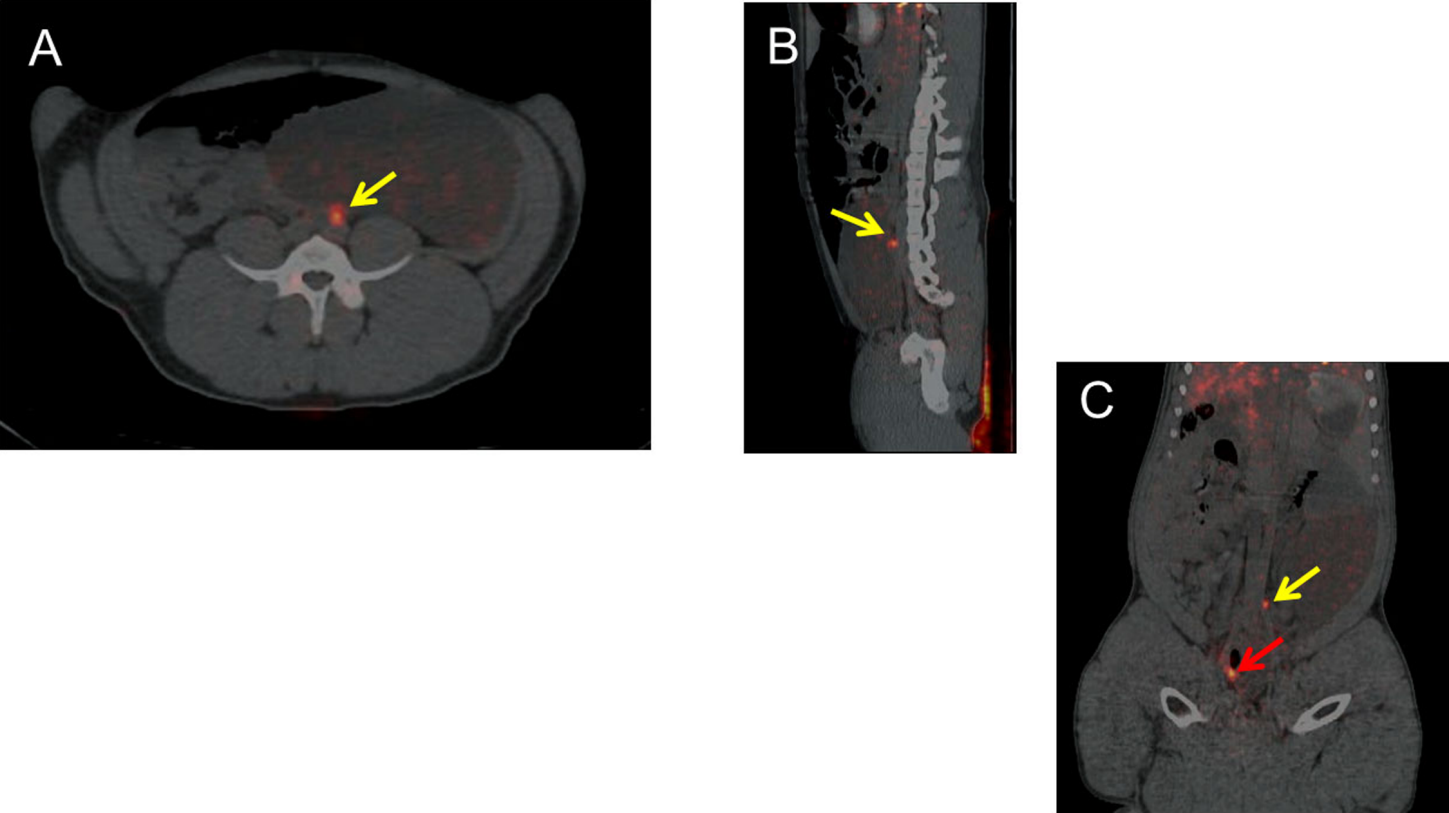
PET/CT imaging ninety minutes after administration of the molecular imaging agent. Fused PET/CT (A) transaxial, (A) sagittal, and (C) coronal views of the Left external iliac node, SUV 11. The injected dose of gallium-68 was 30.0 MBq. At the time of the PET/CT image, the SLN contained 3.0 MBq of gallium-68. There is an additional focus present on coronal view corresponding to the right ureter (red arrow).
